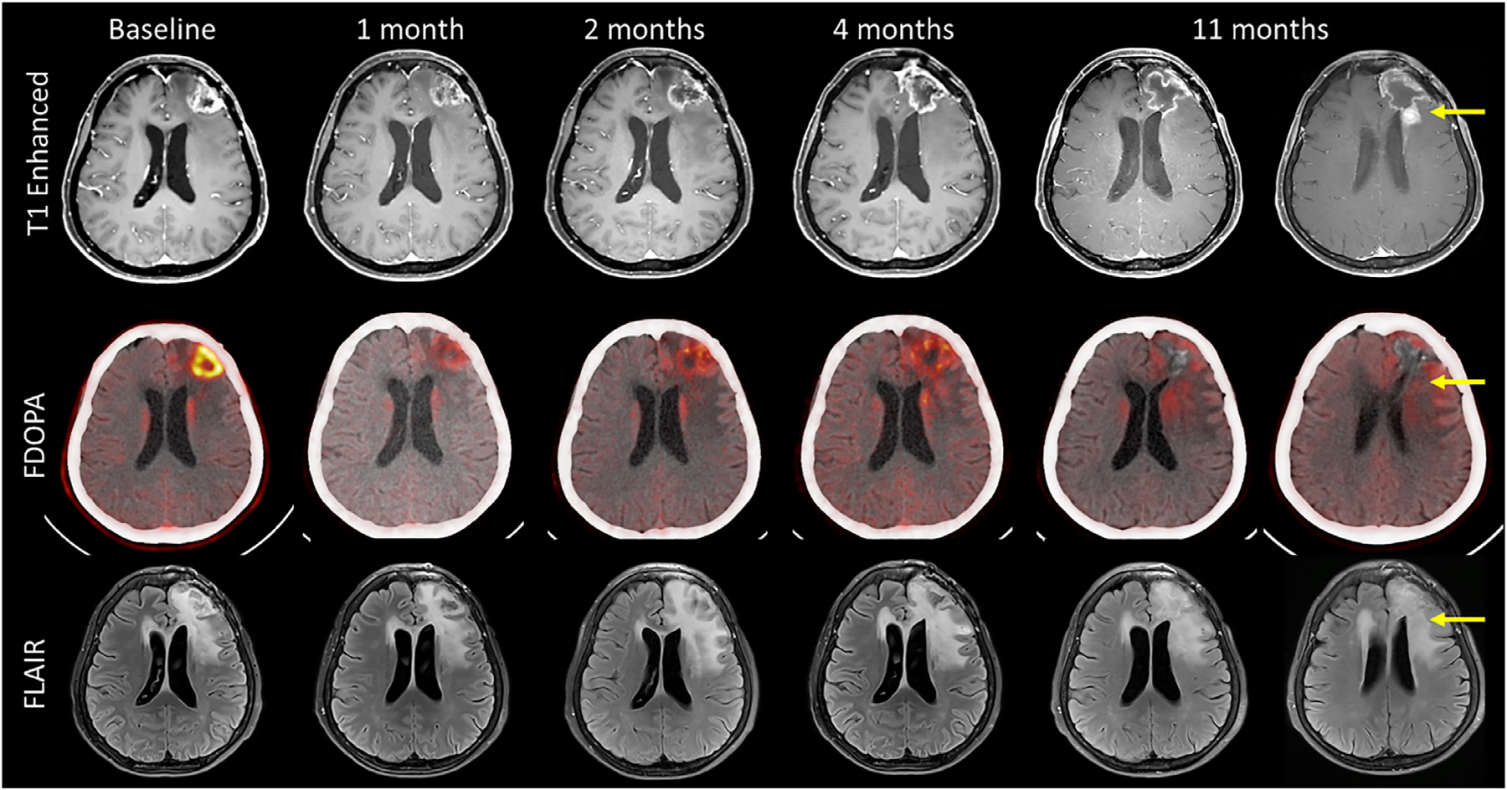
Key example. A 57-year-old female was diagnosed as glioblastoma multiforme with IDH wildtype grade 4, RPA II, MGMT methylated, and TERT-mutant. The patient received tumor resection, radiation therapy, and chemotherapy with temozolomide. Four months after BNCT, a low dose of bevacizumab was administered to control the symptoms of cerebral edema that had increased 2 to 4 months after the BNCT, which accompanies tumor necrosis due to the large tumor size. This case demonstrates the tumor control capability of BNCT, as the patient, despite being a rapidly progressing case with a new 2 to 3 cm tumor detected at the resection margin just 4 months after surgery, remained in a stable condition without evidence of recurrence until 11 months posttreatment. *Abbreviations*: BNCT = boron neutron capture therapy; FDOPA = 6-[18F]fluoro-L-3,4-dihydroxyphenylalanine; FLAIR = fluid-attenuated inversion recovery; IDH = isocitrate dehydrogenase; MGMT = O6-methylguanine-DNA methyltransferase; RPA = recursive partitioning analysis; TERT = telomerase reverse transcriptase.
